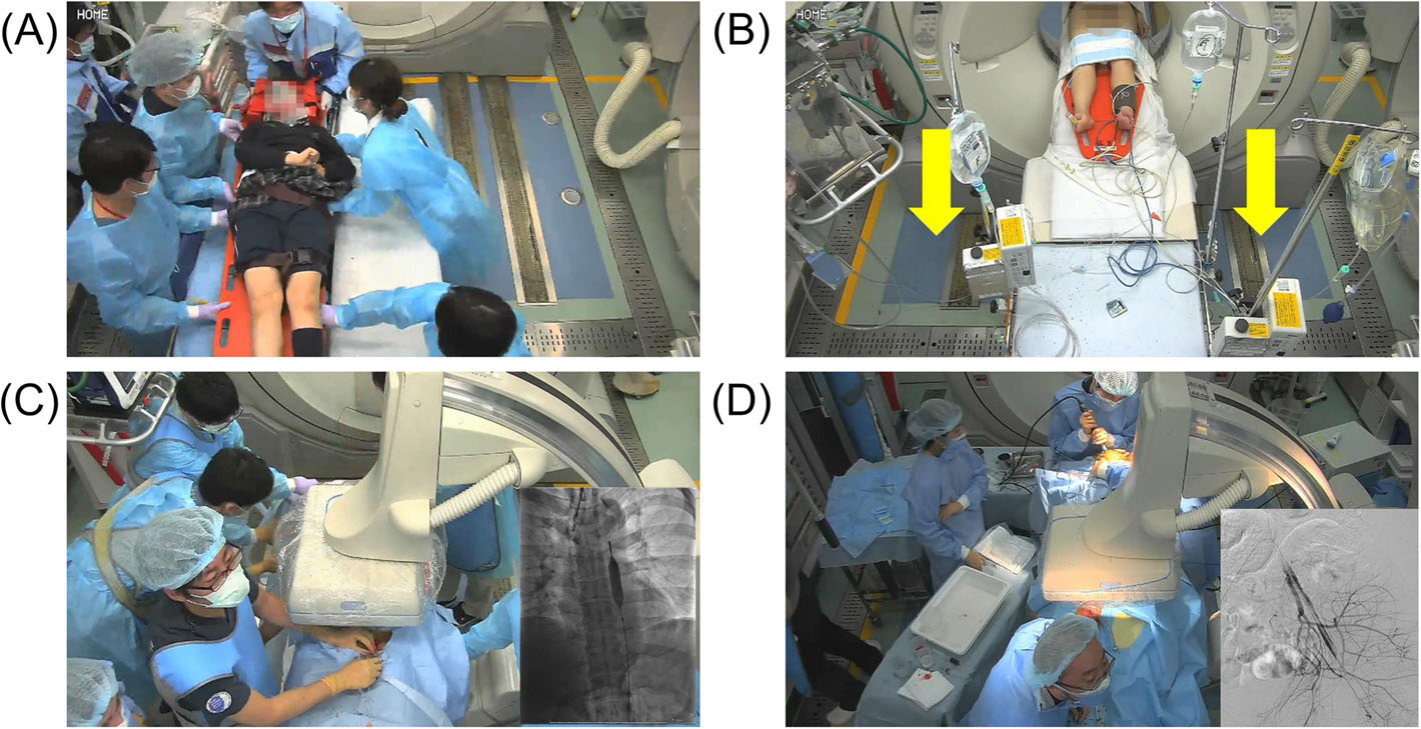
Photographs of the concurrent treatments performed using the HERS. a All doctors and nurses wear radiation protection products before patients’ arrival. Patients are directly accommodated in the hybrid emergency room on arrival. Chest X-ray, pelvic X-ray, and FAST are not performed routinely preceding CT scanning. b After intravenous access is achieved, whole-body CT examination is performed as soon as possible. Head and neck CT are routinely performed without contrast and chest, abdominal, and pelvic CT with contrast. The gantry of the CT scanner moves instead of the patients’ table during CT scanning. c A REBOA catheter is inserted with fluoroscopic guidance to avoid complications. The balloon inflation volume is controlled to maintain a systolic BP of 90–100 mmHg before ICP measurement and to preserve a CPP ≥ 60 mmHg after ICP monitoring. d Bleeding control procedures and ICP monitoring and/or ventriculostomy are performed simultaneously if active torso bleeding and significant intracranial lesion are detected by CT. Neurosurgeons make sure not to interfere with surgical procedures and IR since priority is always given to hemostasis. BP, blood pressure; CPP, cerebral perfusion pressure; CT, computed tomography; FAST, focused assessment with sonography for trauma; HERS, hybrid emergency room system; ICP, intracranial pressure; IR, interventional radiology; REBOA, resuscitative endovascular balloon occlusion of the aorta
